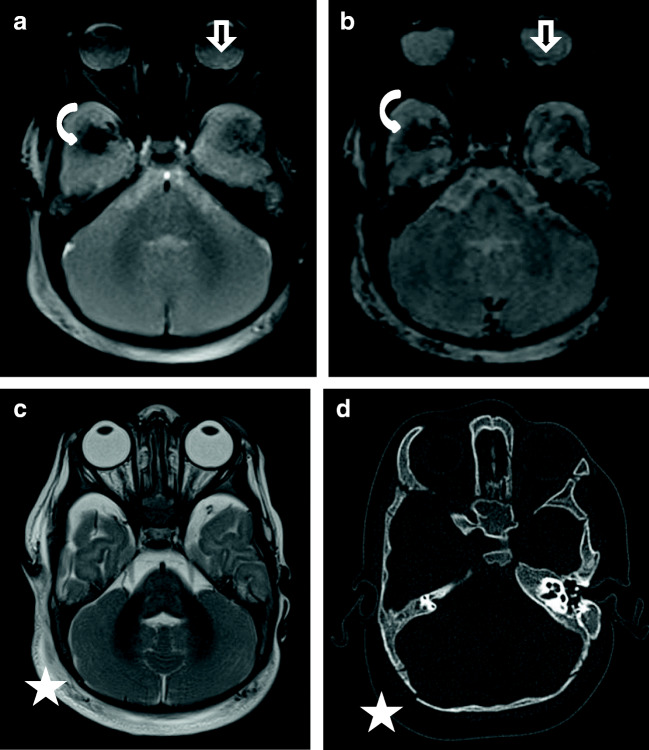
Case “AHT6”. 7 month-old (AHT 6). Presumed AHT with impact (confessed “shaken-impact-syndrome”). 3A: T2* ax, 3B: SWI ax, 3C: T2w ax and 3D: cCT, bone window. Images 3B and 3D were not available to the evaluators. SWI (3B) has a higher sensitivity for the detection of bleeding in MRI. The cortical vein rupture (curved arrow) was better delineated in SWI (3B) compared to T2* (3A). 3A and 3B show a small retinal bleeding (straight arrows). The fracture was difficult to diagnosis in MRI (see T2w:3C) compared to CT (3D). • Anamnesis (not available for the neuro/radiologists): 40 cm high fall on laminate • Due to the additional MRI findings resulting from the impact (fracture and soft tissue swelling) this case was evaluated by one neuroradiologist as an accidental injury. • Two non-neuroradiologists misdiagnosed this case as a NAHT, but did not rule out pAHT. Both investigators recognized evidence of a fracture in the MRI.
